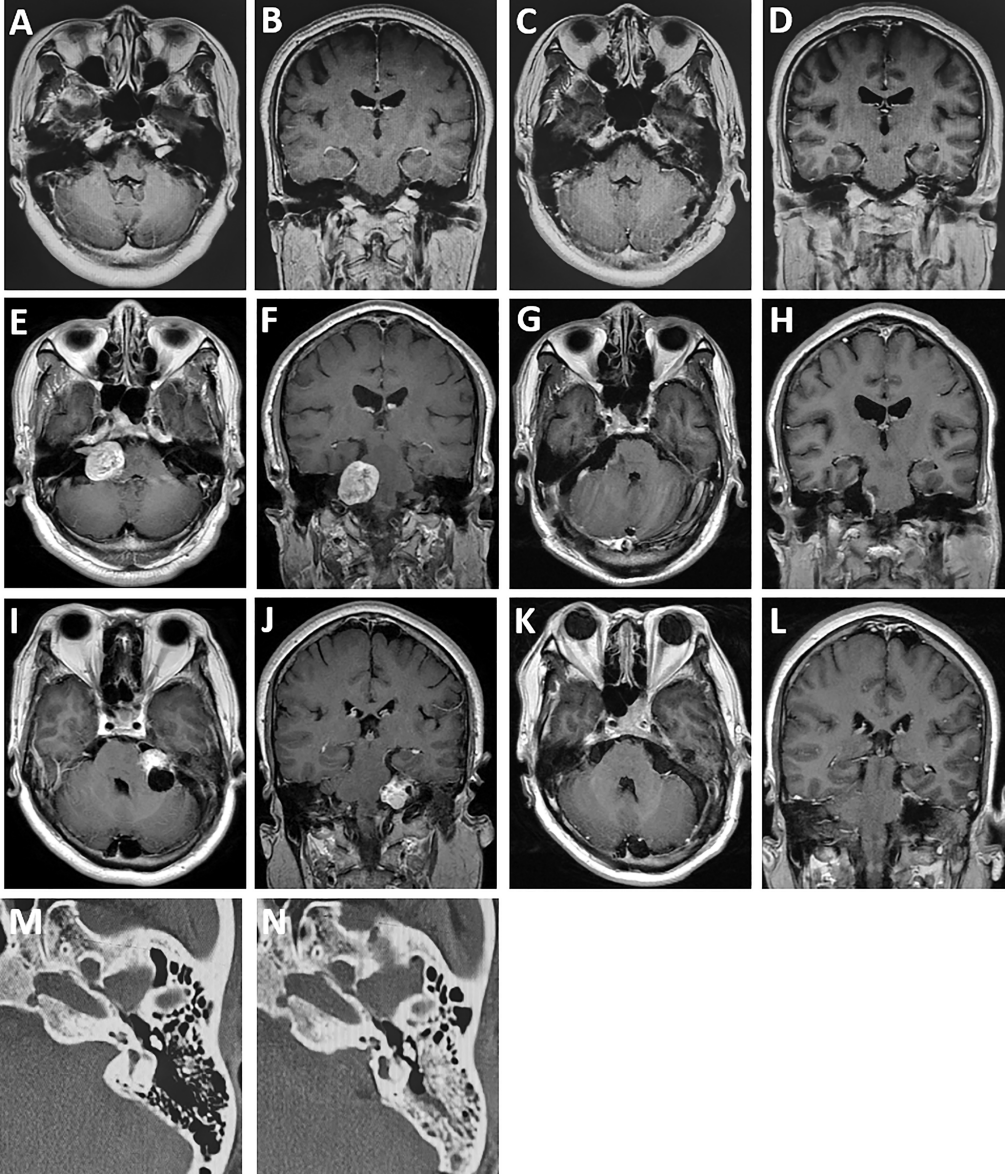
Comparison of preoperative and postoperative MRI. (A, B) A patient with vestibular schwannoma in the internal auditory canal with effective hearing preoperatively. (C, D) MRI at 6-month follow-up revealing total tumor resection and hearing preservation obtained in the patient. (E, F) A patient with solid vestibular schwannoma in the cerebellopontine angle. (G, H) Baseline MRI at 6-month follow-up revealing gross total resection of the tumor and good facial nerve functional preservation (House–Brackmann grade I) after surgery. (I, J) A patient with predominantly cystic vestibular schwannoma with hearing loss before surgery. (K, L) MRI at 6-month follow-up showing no tumor recurrence. (M, N) Comparison of the bone window CT scans of the internal auditory canal before and after grinding.
